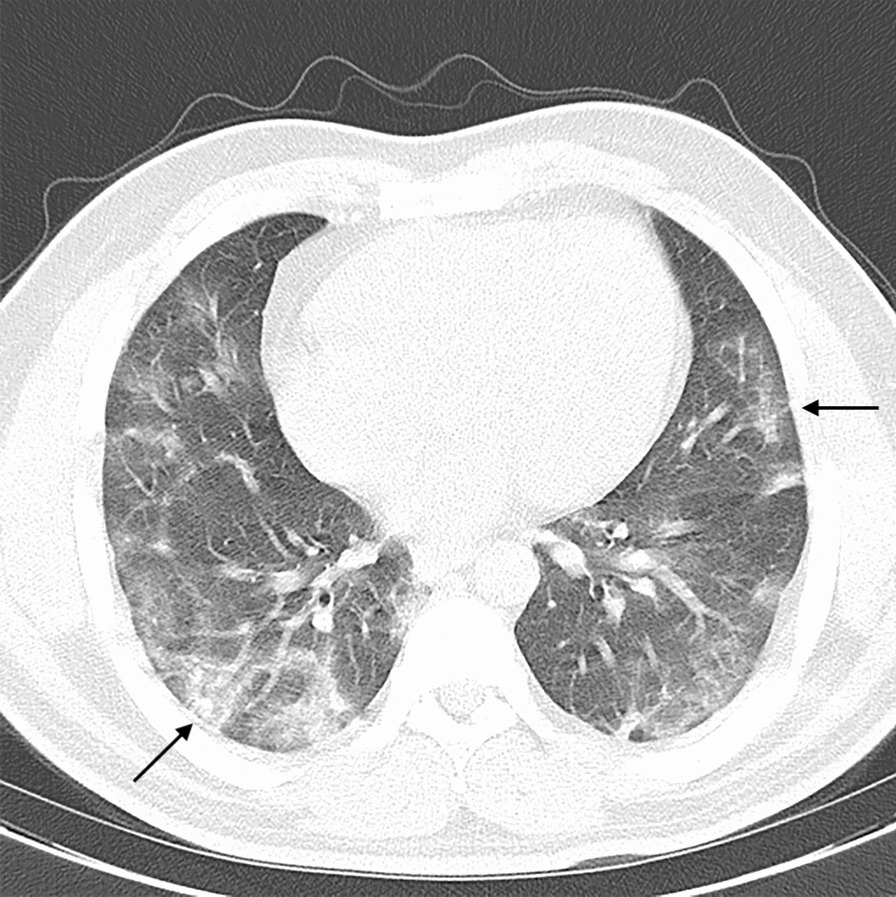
Chest CT scan shows bilateral multifocal subpleural and peribronchial ground-glass opacities (black arrows) compatible with COVID-19 disease
